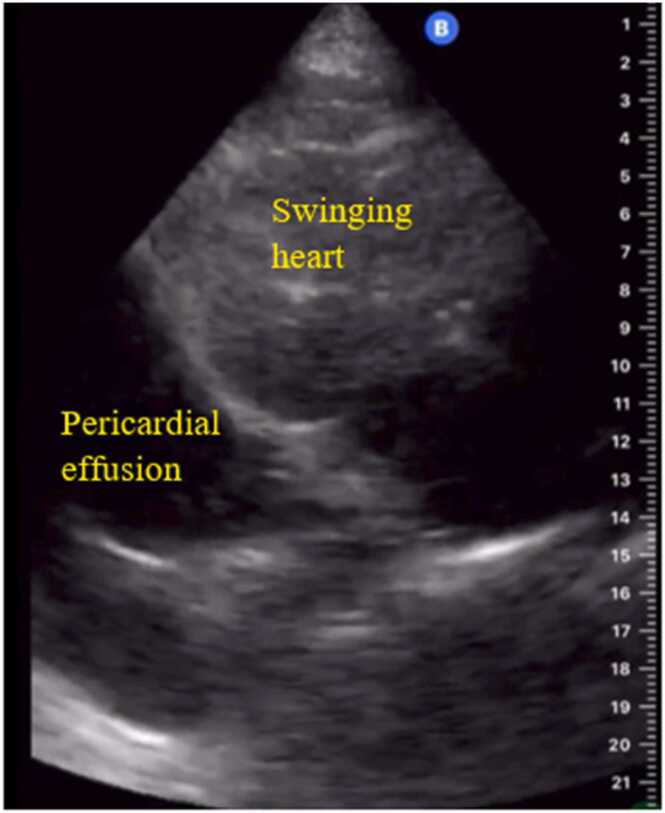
Representative of ultrasound image findings for obstructive shock; large pericardial effusion with swinging heart.
